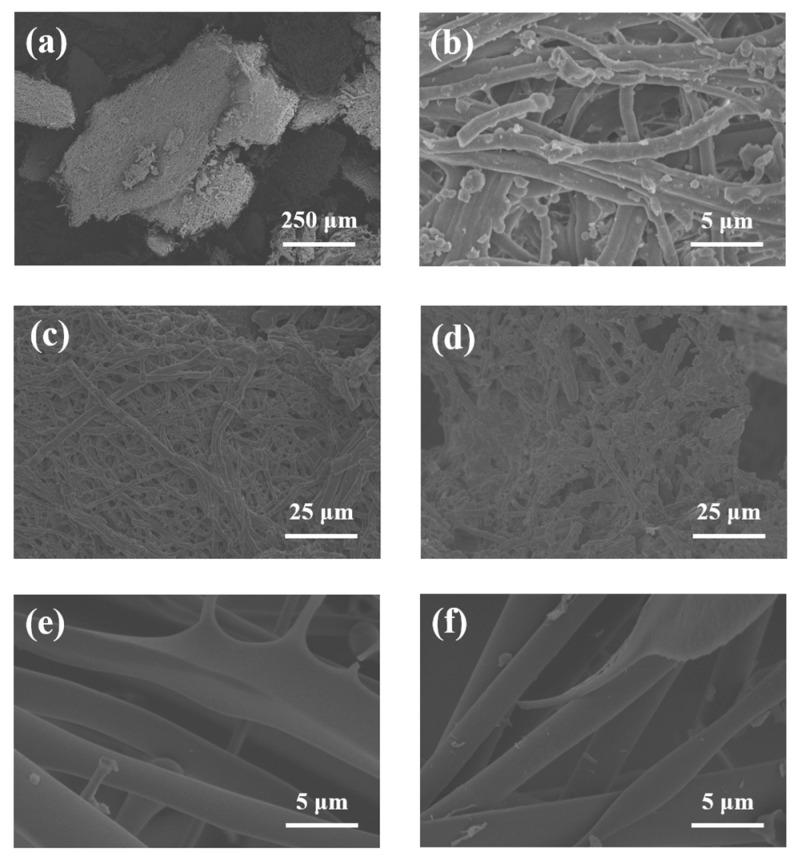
SEM image of different samples. (a,b) ESM; (c) ESM with enzyme treatment; (d) ESM with ultrasonic-assisted enzyme treatment; (e) PSC, and (f) UPSC.
